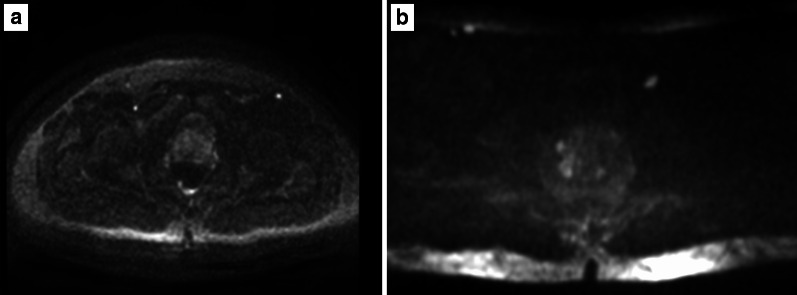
DWI of a suboptimal (38 × 40 cm) (a) and optimal (17 × 20 cm) (b) field of view according to the PI-RADS v.2.1 guidelines
